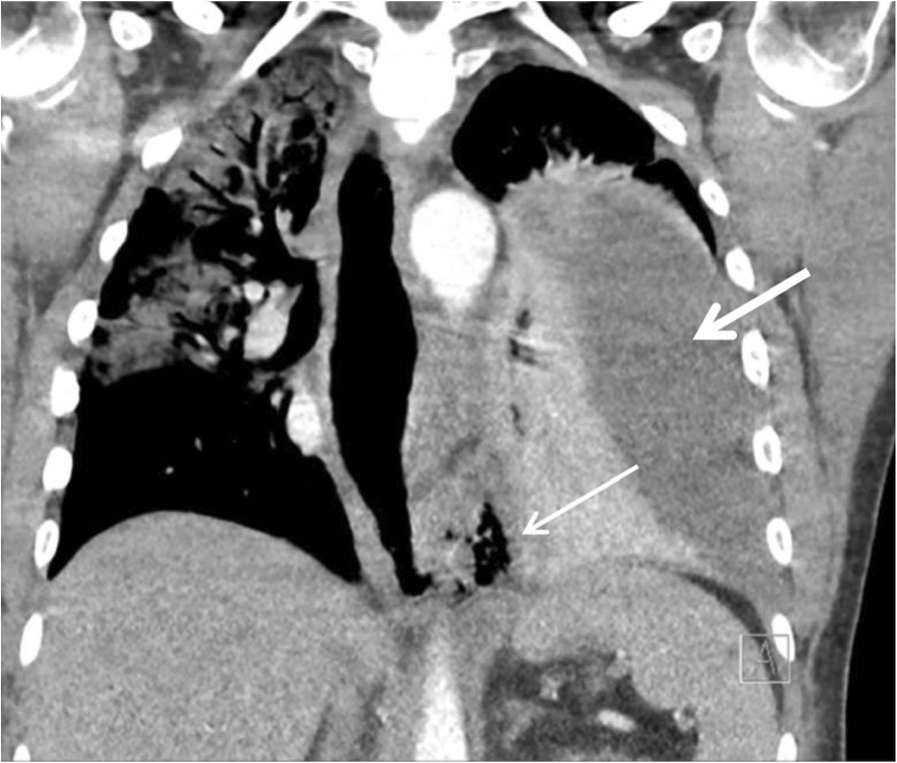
Computed tomography pulmonary angiogram demonstrating a dilated lower oesophagus with a defect at its lateral aspect with gas extending into the left pleural space (thin arrow), consistent with perforation. This is associated with a large left pleural effusion (thick arrow). Coronal view
